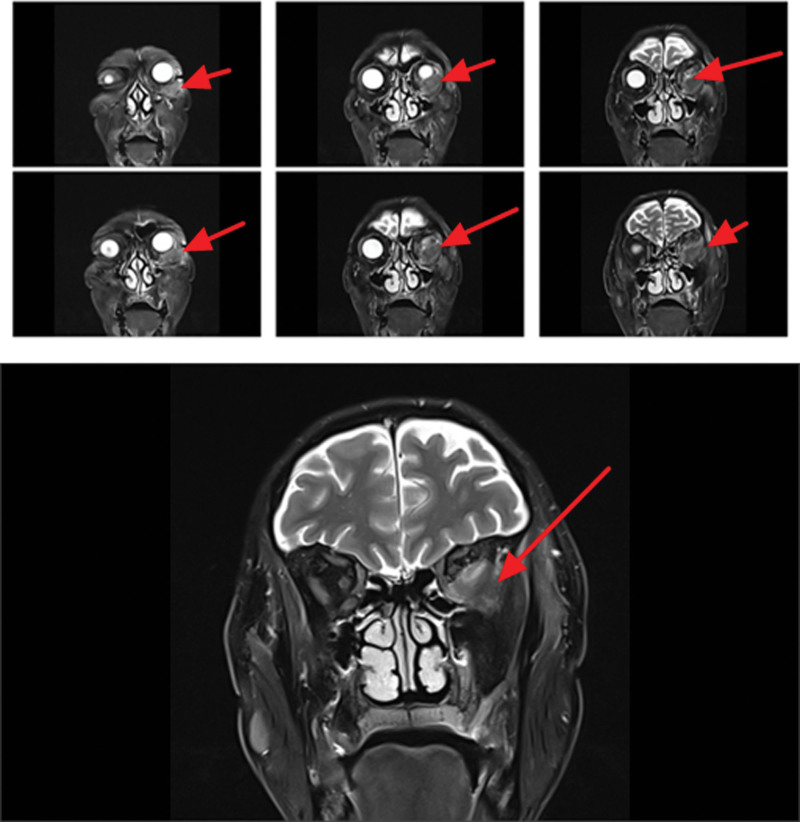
The patient MRI coronal T2 phase left posterior ocular fat space, left lateral ocular, and left infraocular muscular space masses showed slightly high signal (red arrows). MRI = magnetic resonance imaging.
